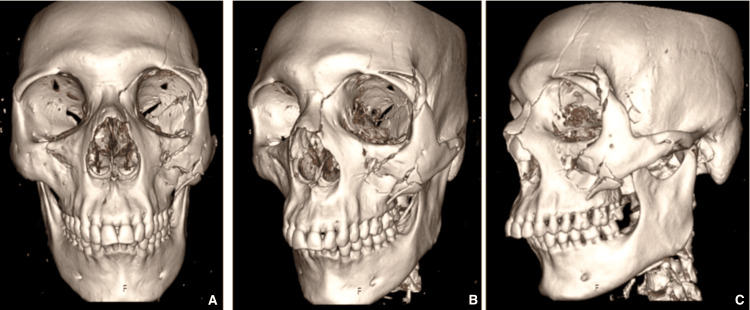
Preoperative CT imaging - 3D reconstruction. The first image (A) corresponds to the anterior-posterior view; the second image (B) corresponds to the left oblique view; and the third image (C) corresponds to the left lateral view.
